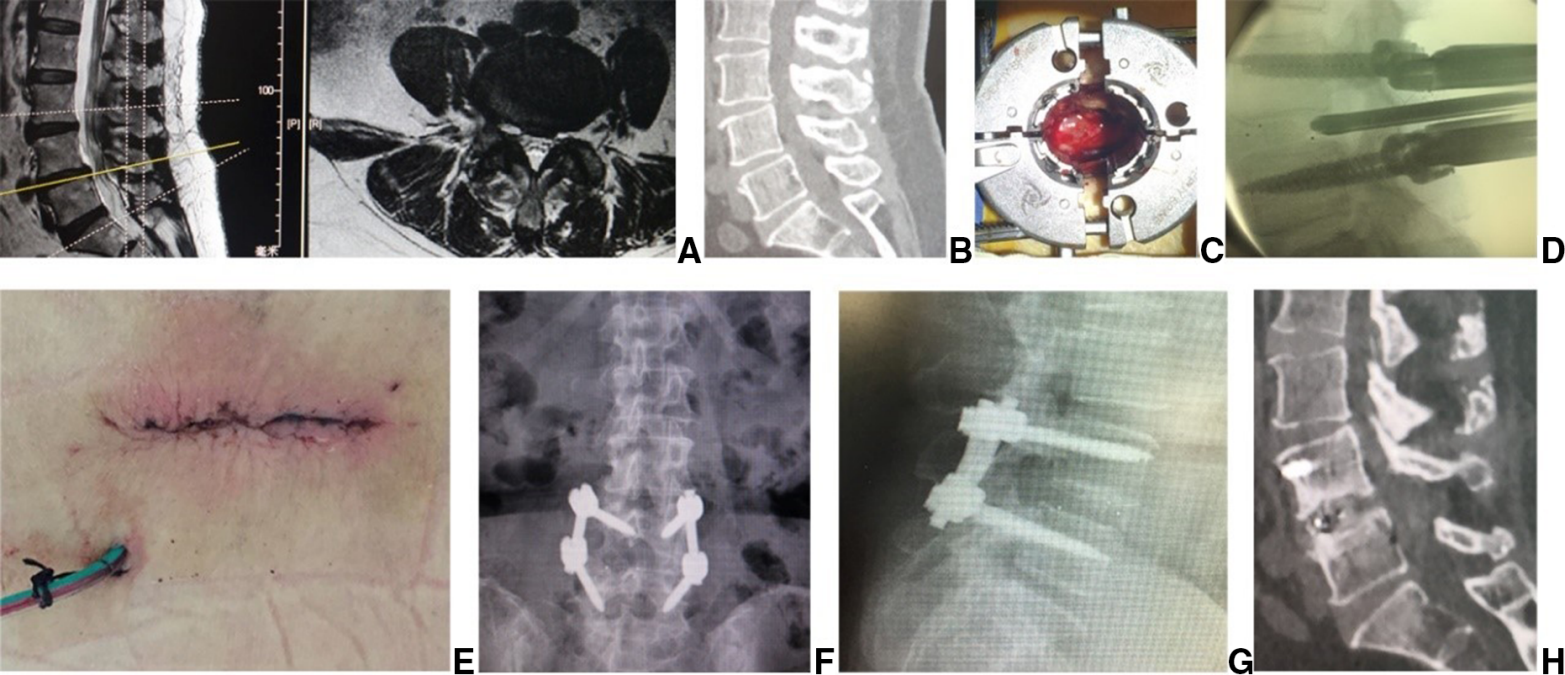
Female patient of 72 years with LDD at L4/5 had right leg pain. Preoperative (A) sagittal, axial MR and (B) CT images showed L4/5 disc herniation, lateral recess stenosis and neurologic compression. In MB-TLIF, (C) through an expandable tubular retractor, the dun and nerve root involved were exposed after a unilateral complete facetectomy and hemilaminectomy. (D) The fluoroscopic image showed that a cage filled with autograft bone was inserted after the disc material and cartilaginous endplate were removed. Two rods were fixed over pedicle screws and (E) the wound was closed in layers with the drainage tube placed. (F,G) Postoperative x-ray confirmed that the position of pedicle screws and cage was good. Fusion grade based on the Bridwell grading system at 2 year follow-up WU Grade I on (H) sagittal CT.
